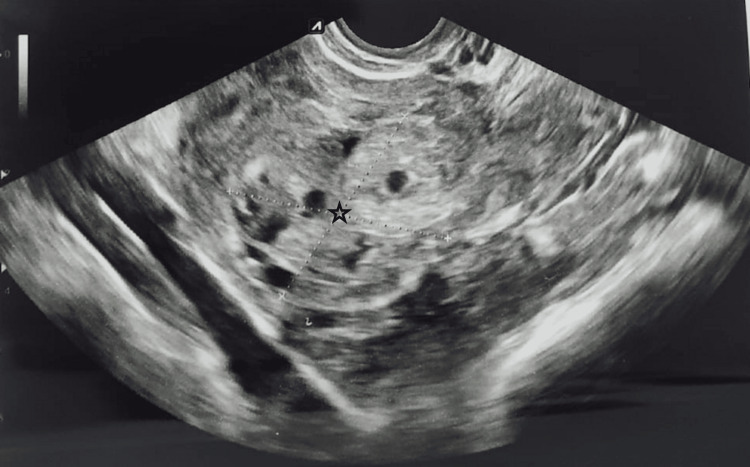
Ultrasonography shows increased vascularity and enlargement of the right adnexa (indicated by the star mark).
